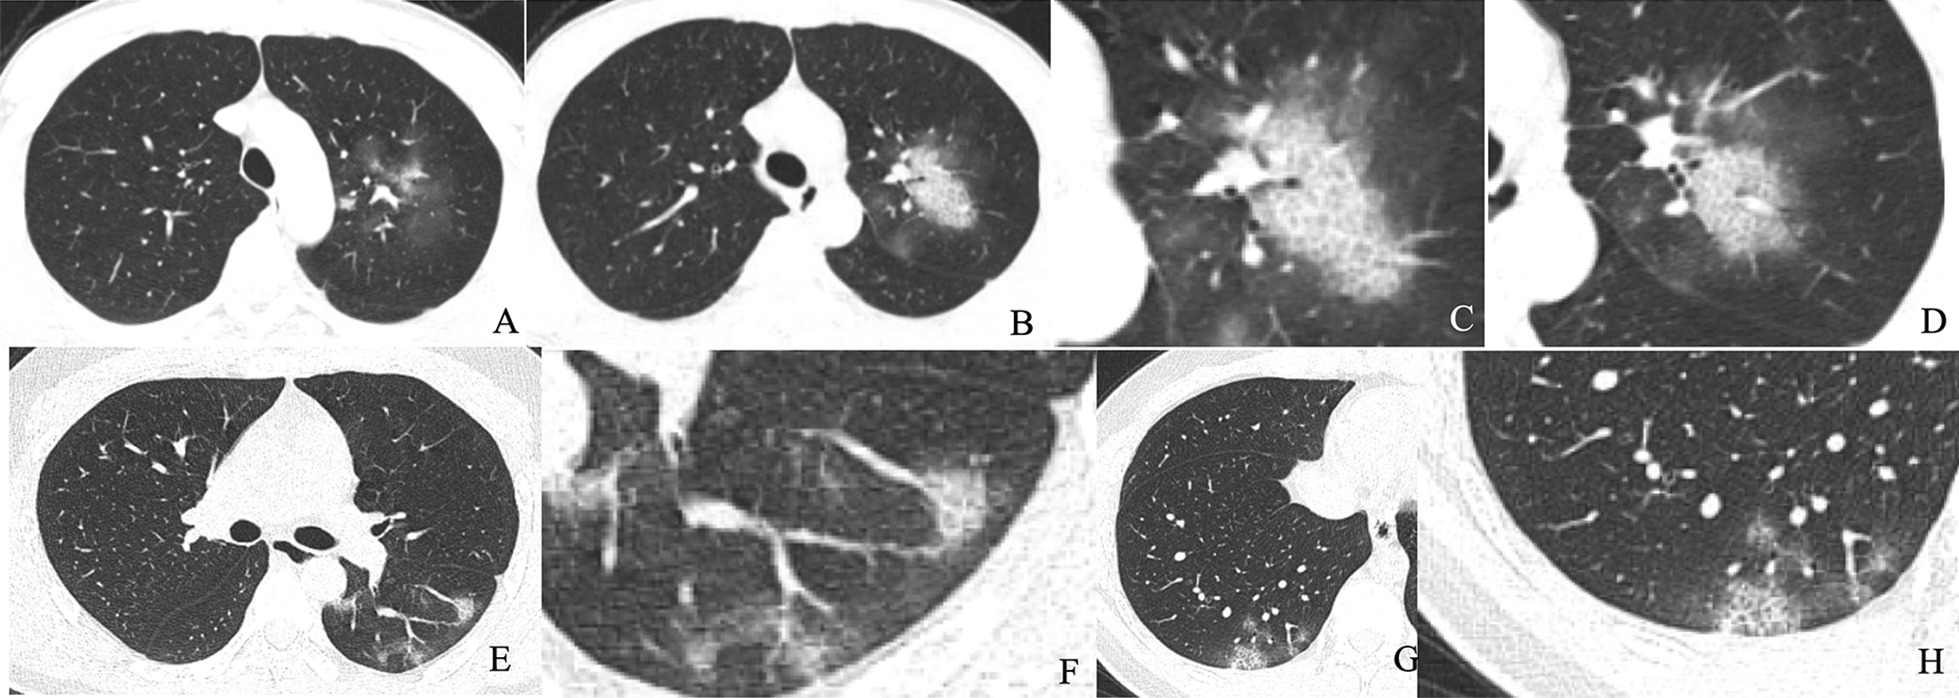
Chest CT findings of COVID-19 pneumonia. a–d Male, 28 years old, 3 days after onset, axial view by CT scan. Multiple pure GGO in the upper left lobe (a, b), intralobular interstitial thickening and halo sign (c), vascular thickening and halo sign (d). E–H: Male, 25 years old, 2 days after onset, axial view by CT scan. Multiple pure GGO in the lower of both lobe (e, g), vascular thickening and halo sign (f); intralobular interstitial thickening and halo sign (h)
